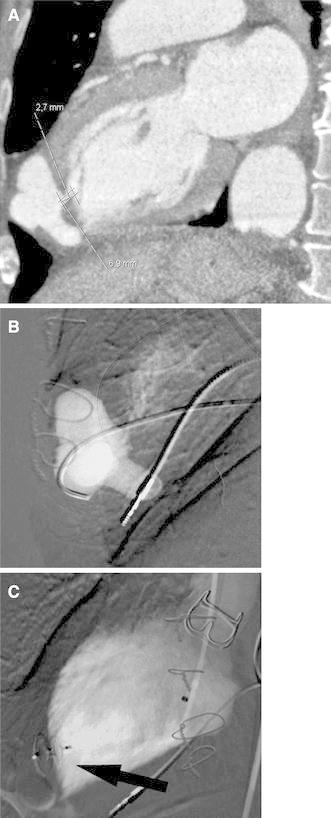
Apical pseudoaneurysm. A CT showing apical pseudoaneurysm. B Catheter positioned in the pseudoaneurysm at angiography. C Exclusion of the pseudoaneurysm by amplatzer septal occluder (*arrow*)
